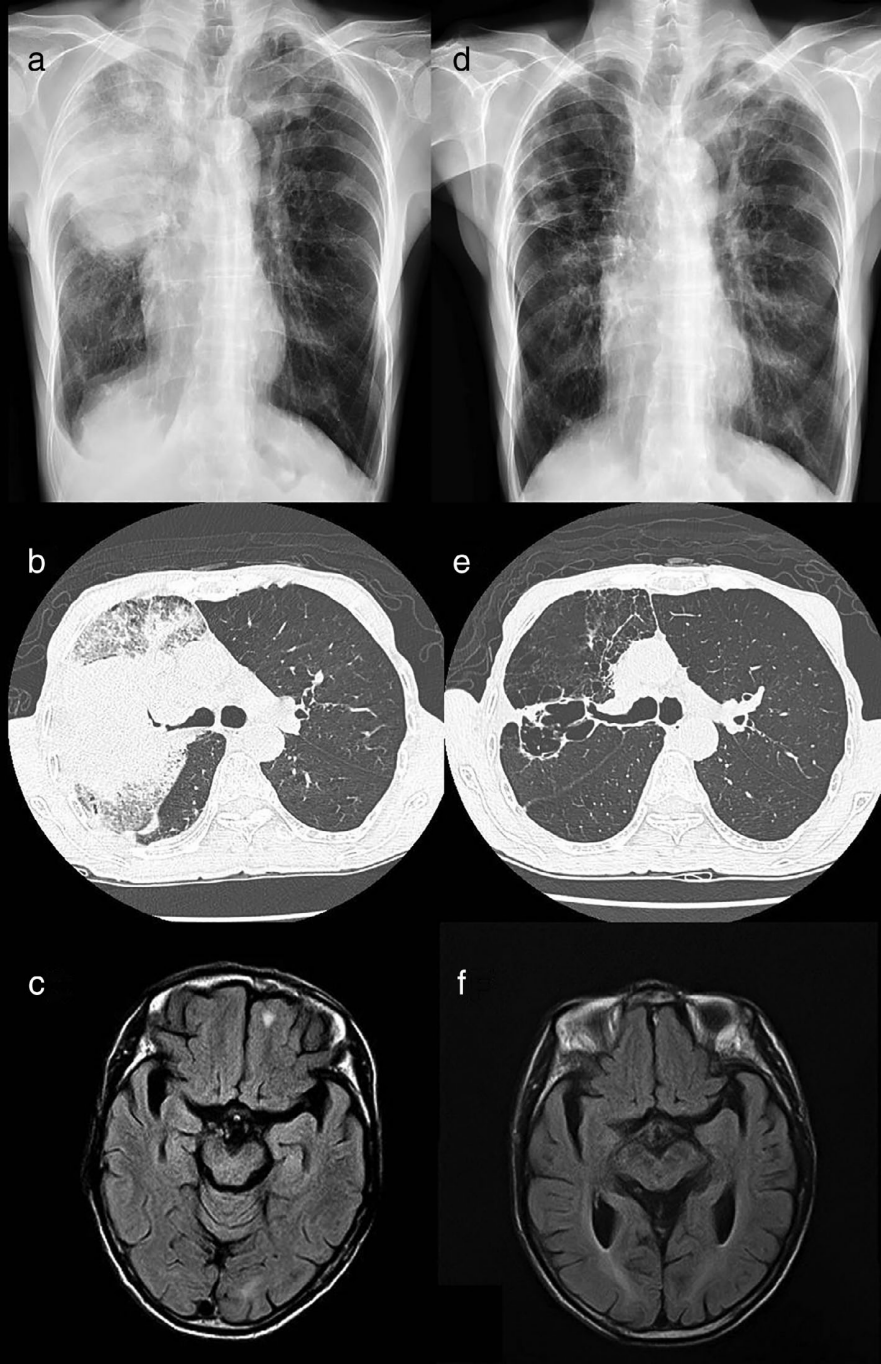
Findings of chest radiograph, computed tomography (CT), and brain magnetic resonance imaging (MRI) performed before and after the administration of combination therapy. (a) A huge mass in the right upper lung (RUL) with right pleural effusion was observed on the chest radiograph. (b) A huge mass in the RUL of up to 10.6 cm in diameter with necrotic components and narrowing of the RUL bronchus was visible on chest CT. (c) One metastatic tumor was observed in the left inferior frontal lobe before the administration of combination therapy. (d) The size of the RUL mass significantly decreased, and no pleural effusion was found on chest radiograph. (e) The primary RUL mass had significantly decreased in size and exhibited multiple cavities on chest CT. (f) Complete remission of the metastatic tumor in the left inferior frontal lobe was observed after administration of combination therapy
